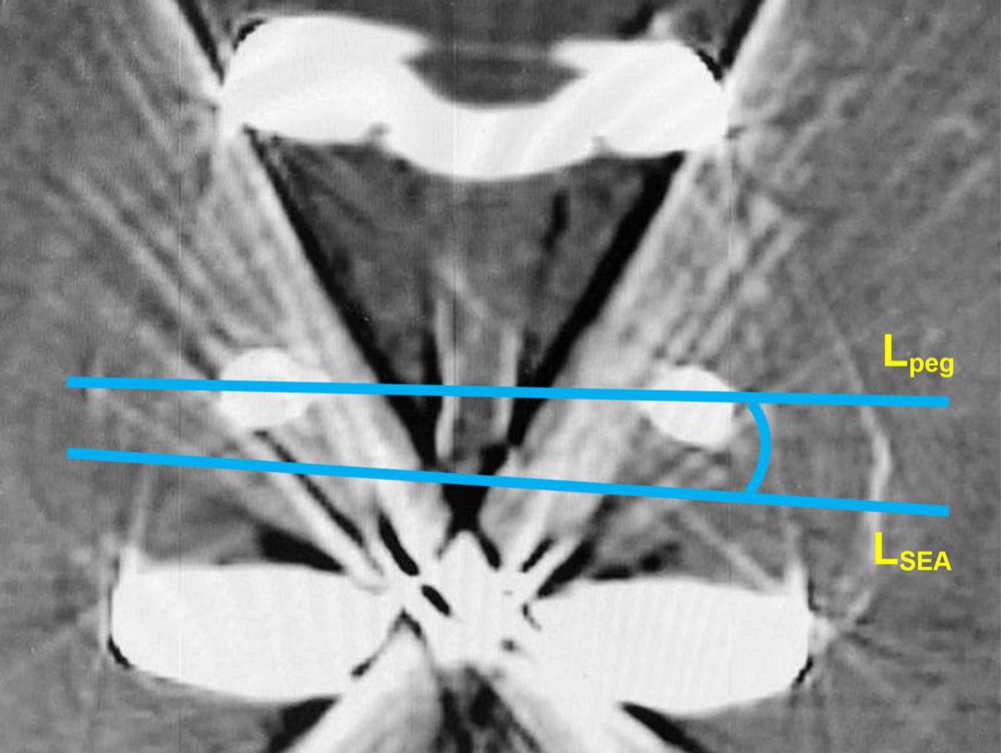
Method for measuring femoral component rotation using computed tomography. Femoral component rotation was evaluated on the axial slice where two femoral pegs and the medial and lateral epicondyles were visible. Femoral rotation was the angle between the line connecting the two pegs (*L*
